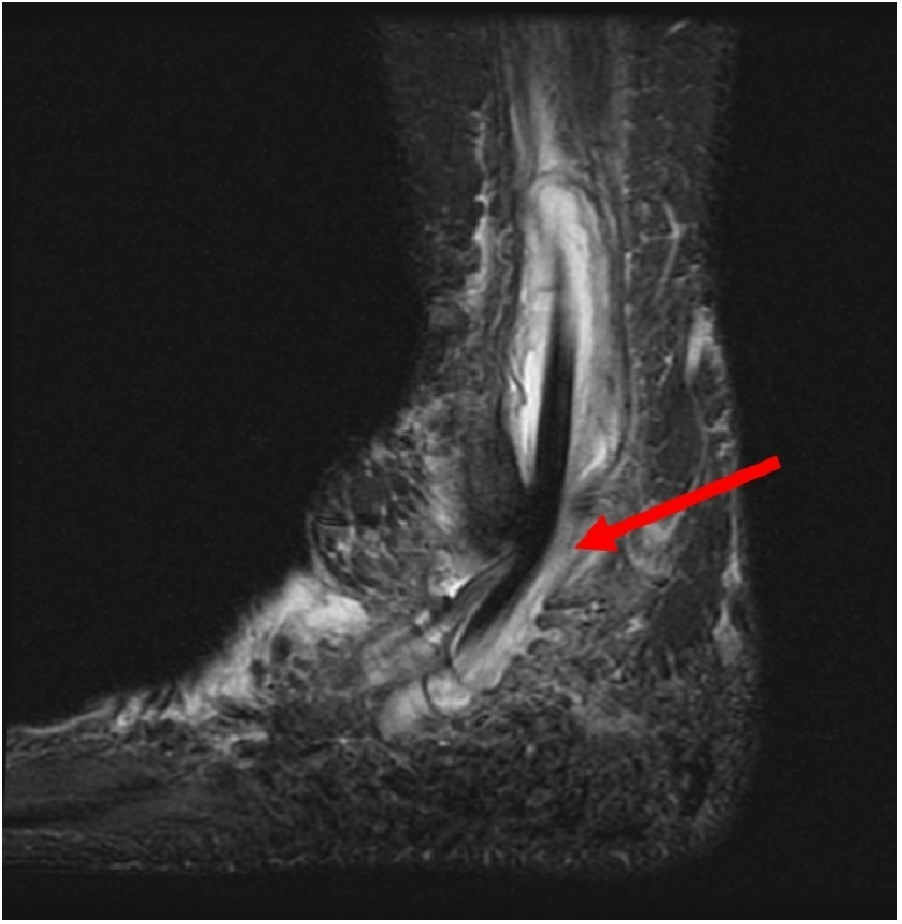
Lateral 3T MRI of the right foot. Marked fluid distention and synovial thickening distend the common peroneal tendon sheath and peroneal tendons (arrow). MRI: Magnetic resonance imaging.
